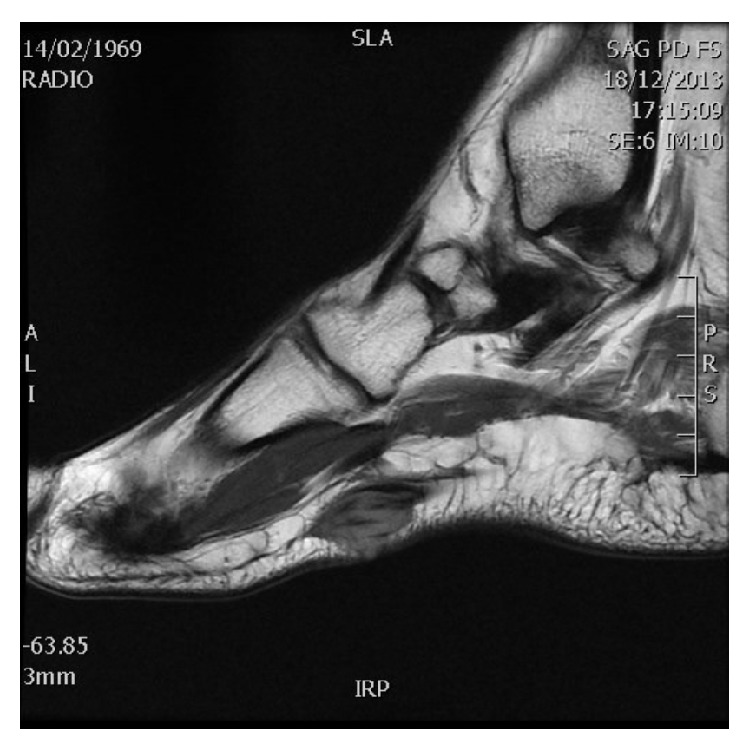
MRI of the right feet: sagittal T1-weighted image demonstrated a nodular soft-tissue masse in the distal plantar fascia, which are hypointense to muscle.
